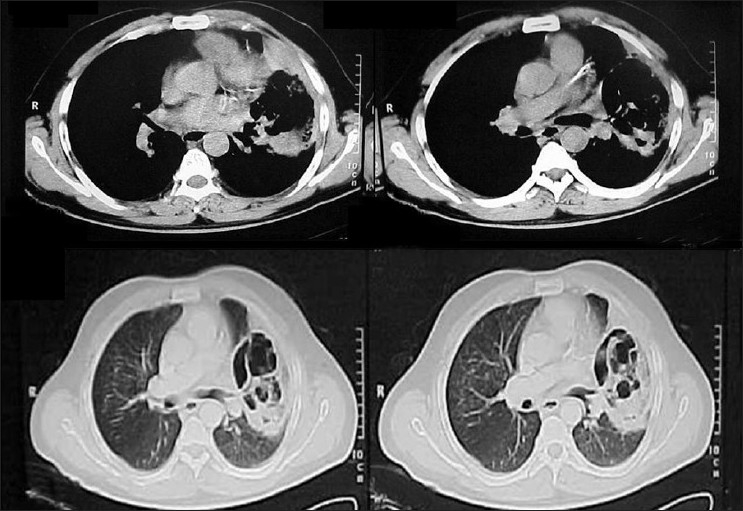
CT chest shows soft tissue attenuating lesion with multiple cavities involving left upper lobe and lingula
